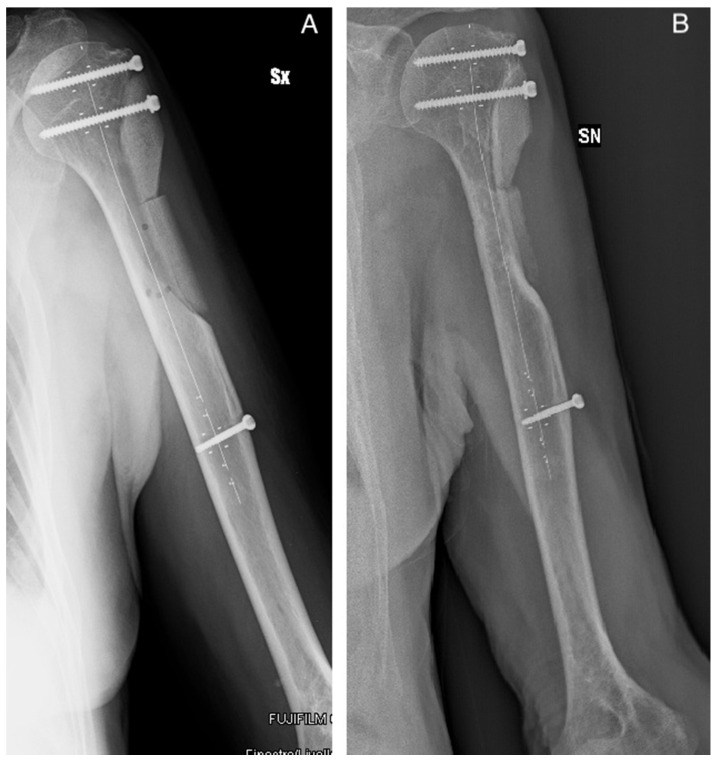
Patient 18. Radiological control after one month from Myxofibrosarcoma excision with (A) lateral proximal humerus cortical resection and reconstruction with two SB blocks. (B) The radiograph on the right shows a line of radiolucency and a partial resorption of the lowest block due to the incomplete coverage of the graft and its isolation from the soft tissues. Abbreviations: SX and SN stand for Left.
